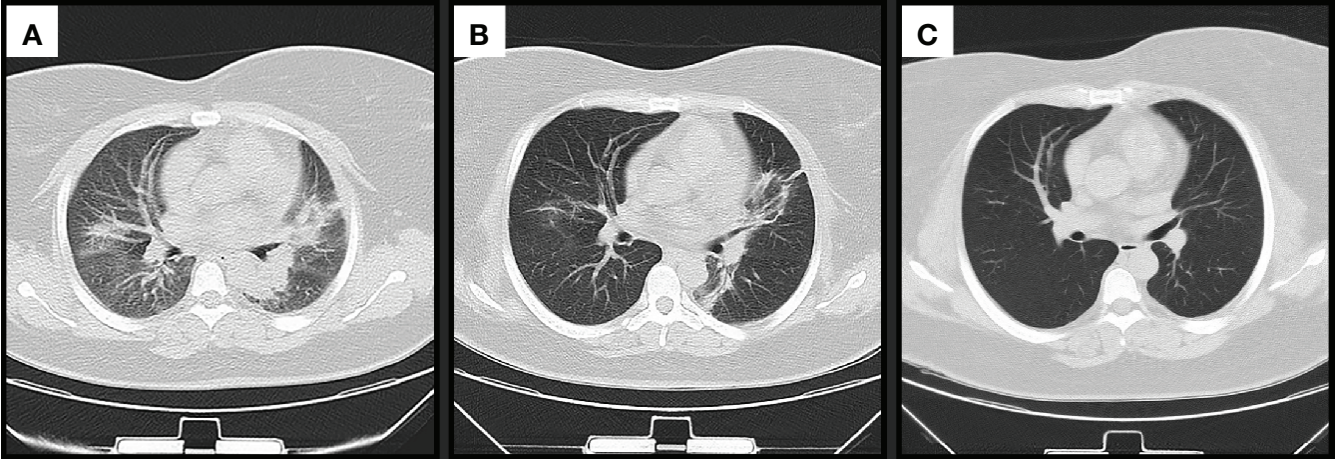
Changes in chest computed tomography scan findings (A) on admission, (B) before discharge and (C) after recovery.
